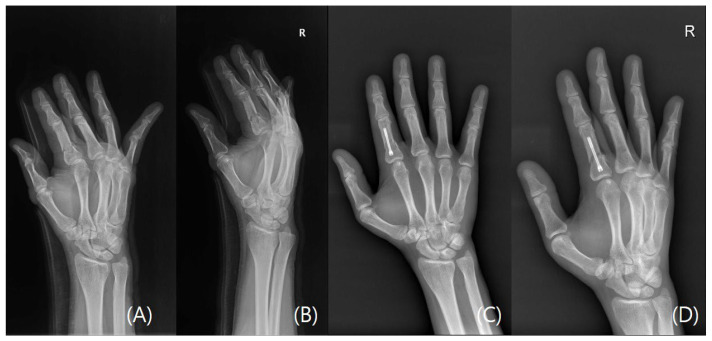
A case of a right second digit transverse proximal phalangeal fracture. (A) Preoperative plain radiograph Posteroanterior view. (B) Preoperative plain radiograph oblique view. (C) Postoperative plain radiograph Posteroanterior view taken at last follow-up visit. (D) Postoperative plain radiograph oblique view taken at last follow-up visit. The letter R refers to the right hand.
